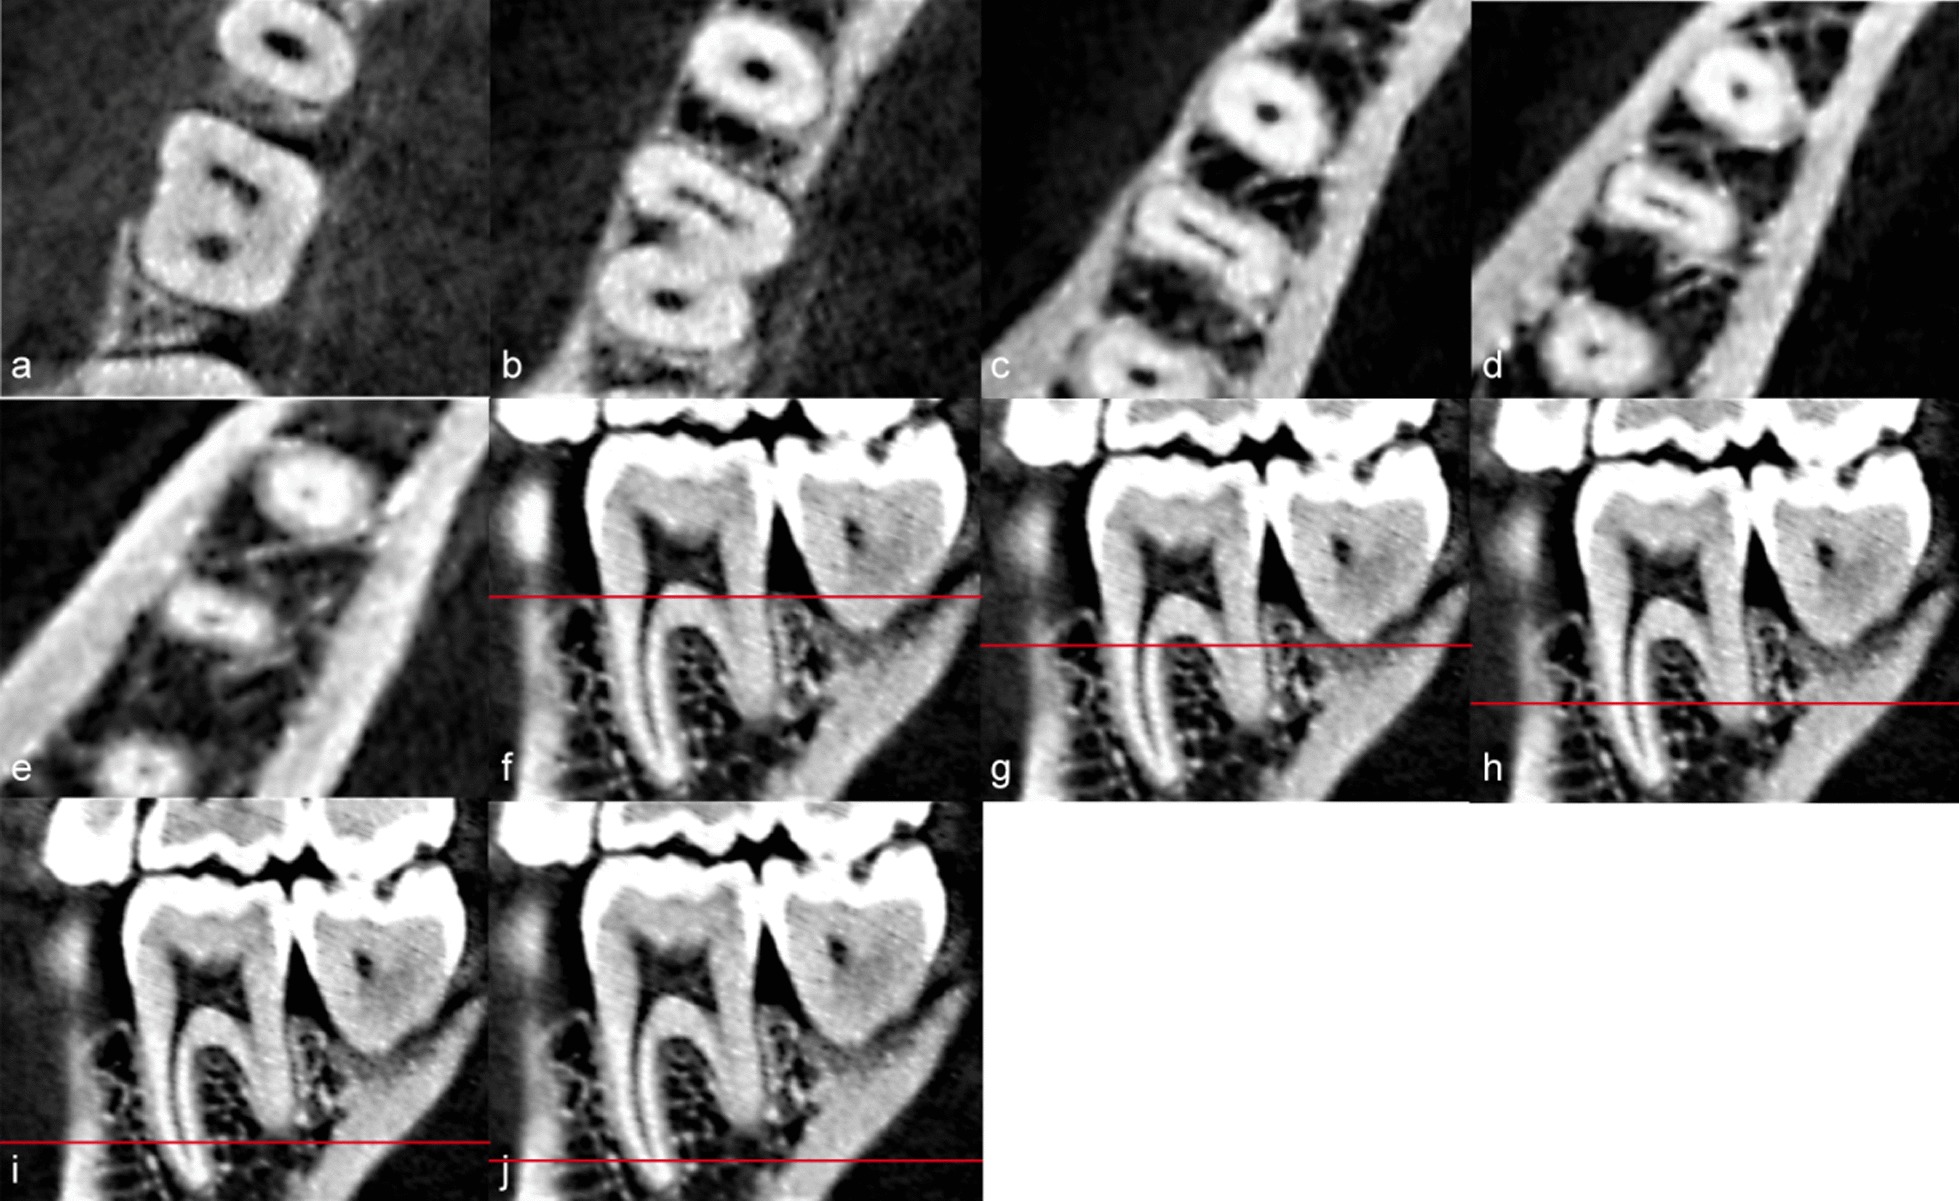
CBCT images of the 3-4-3-2-1 root canal system type. a–e were CT images with different cross sections: This type is more complicated, there are three root canals at the bottom of the pulp chamber, then four branches are branched, and fused into three root canals, then two canals can be seen at apical 1/3 of root canal. The root canals eventually fused into one apical foramen; f–j sagittal images
